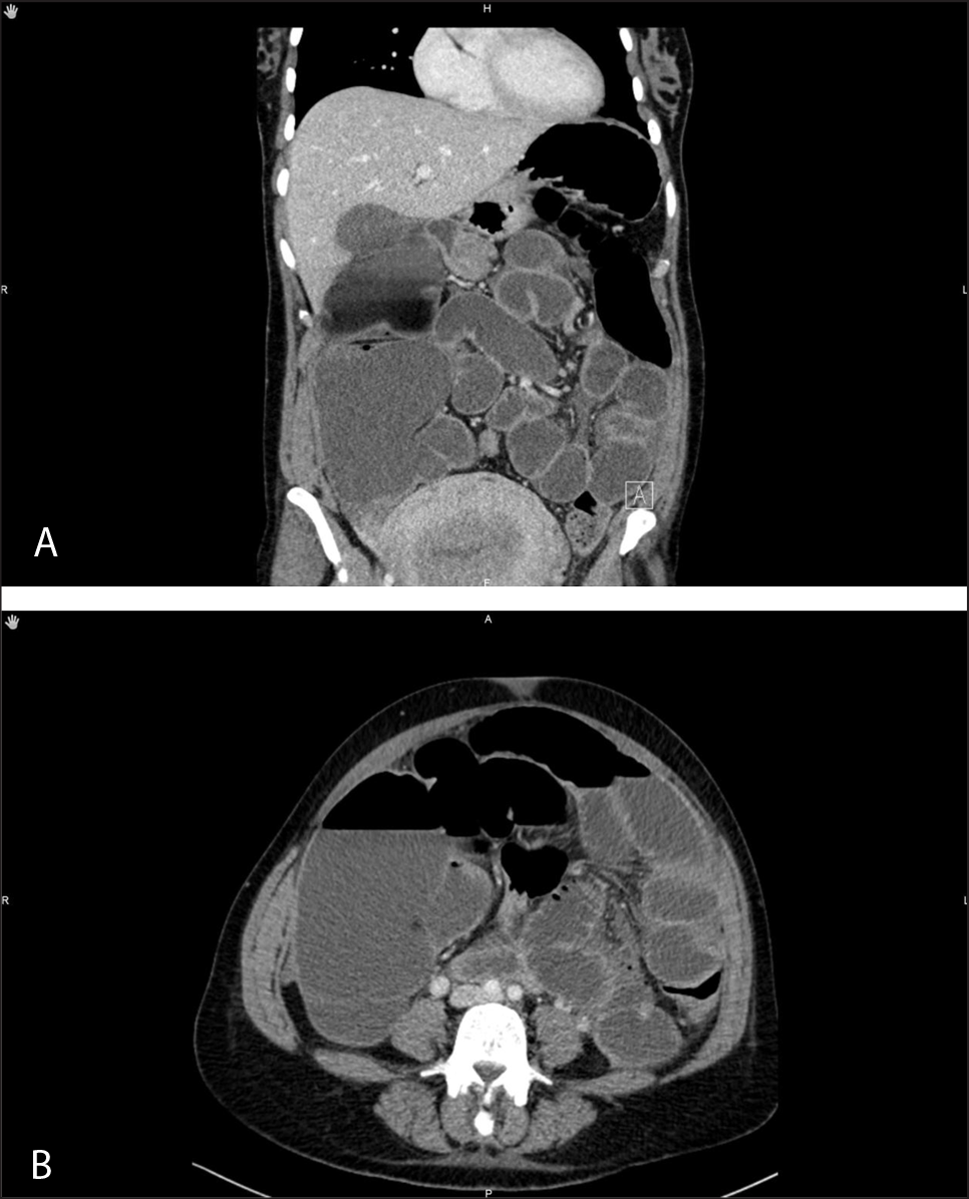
Abdominal contrast-enhanced CT revealing distension (with air-fluid levels) of the stomach, the small bowel and both ascending and transverse colon.
